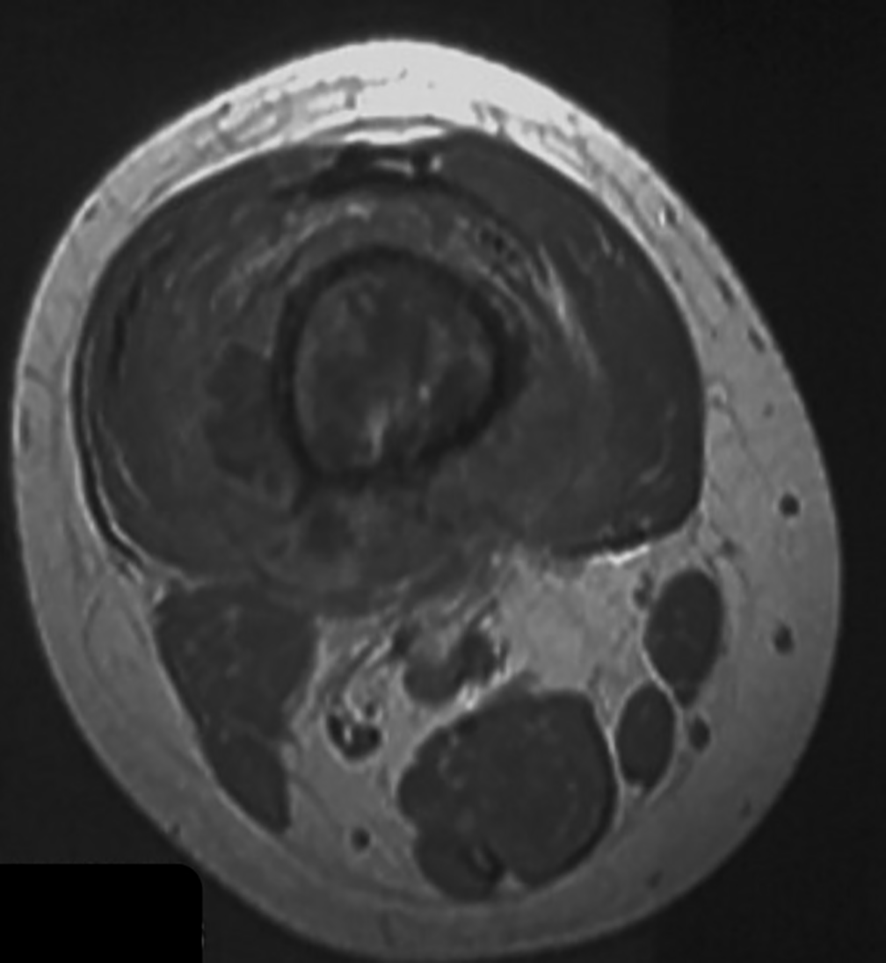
A T1-weighted image of a distal femur indicating a poor response to neoadjuvant chemotherapy.
